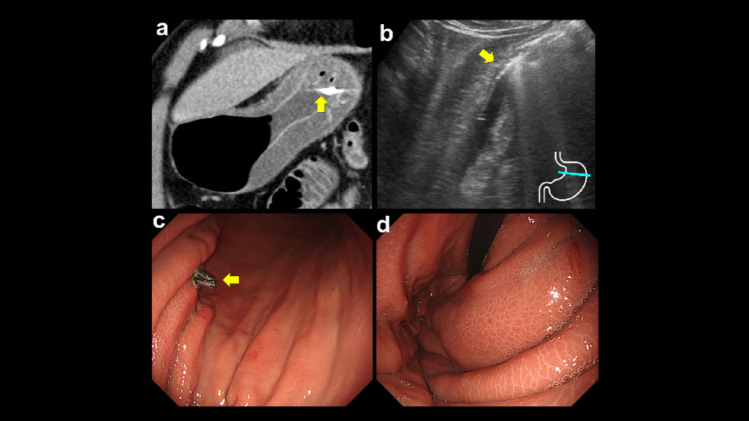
a Contrast-enhanced computed tomography (CT) the day after endoscopic mucosal resection (EMR) showed segmental circumferential gastric wall thickening from the fundus to the body at the clipped site (yellow arrow), suggesting phlegmonous gastritis (PG). b Ultrasonography confirmed circumferential thickening with preserved layers and marked submucosal edema (the yellow arrow indicates the clip placed during EMR). c Endoscopy on day 6 showed gastric body wall thickening near the resection site closed by clipping (yellow arrow). d Similar mucosal changes in the fornix indicated healing phase of PG
